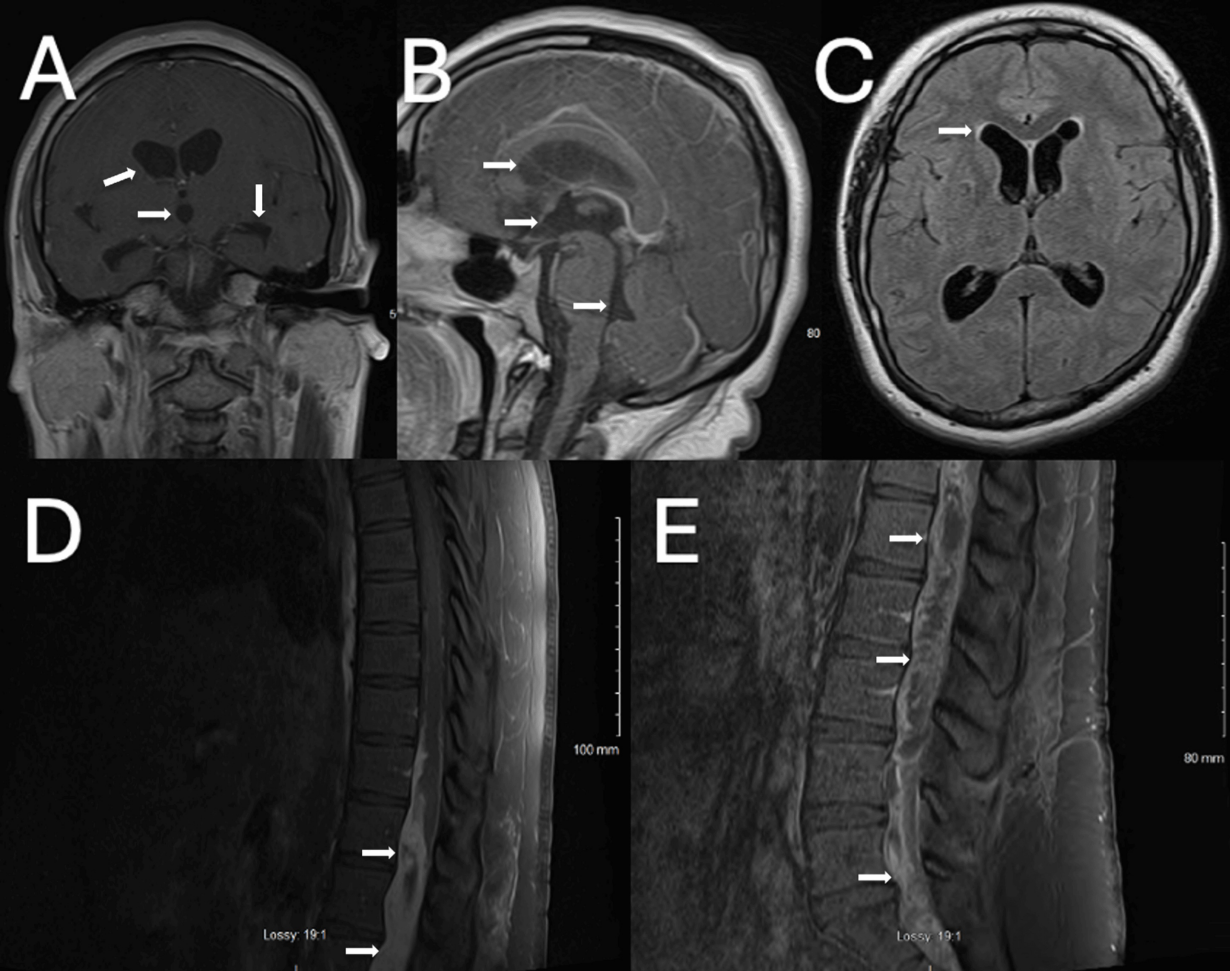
(A) Preoperative computed tomography (CT) of the head without contrast shows enlarged lateral ventricles, temporal horns, and third ventricle (white arrows). (B) Preoperative magnetic resonance imaging (MRI) of the brain without contrast again shows lateral ventricle, third ventricle, and fourth ventricle ventriculomegaly (white arrows) without any obstructive lesions, suggesting communicating hydrocephalus. (C) Preoperative MRI brain T2 FLAIR does not show transependymal flow (white arrow). (D-E) Preoperative MRI thoracic and lumbar spine T1 with contrast show an intradural intramedullary heterogeneously enhancing lesion spanning from T10 to S2 (white arrows) with compression on the spinal cord, conus medullaris, cauda equina, and filum terminale with invasion of neural foramina at multiple levels. FLAIR: fluid-attenuated inversion recovery.
